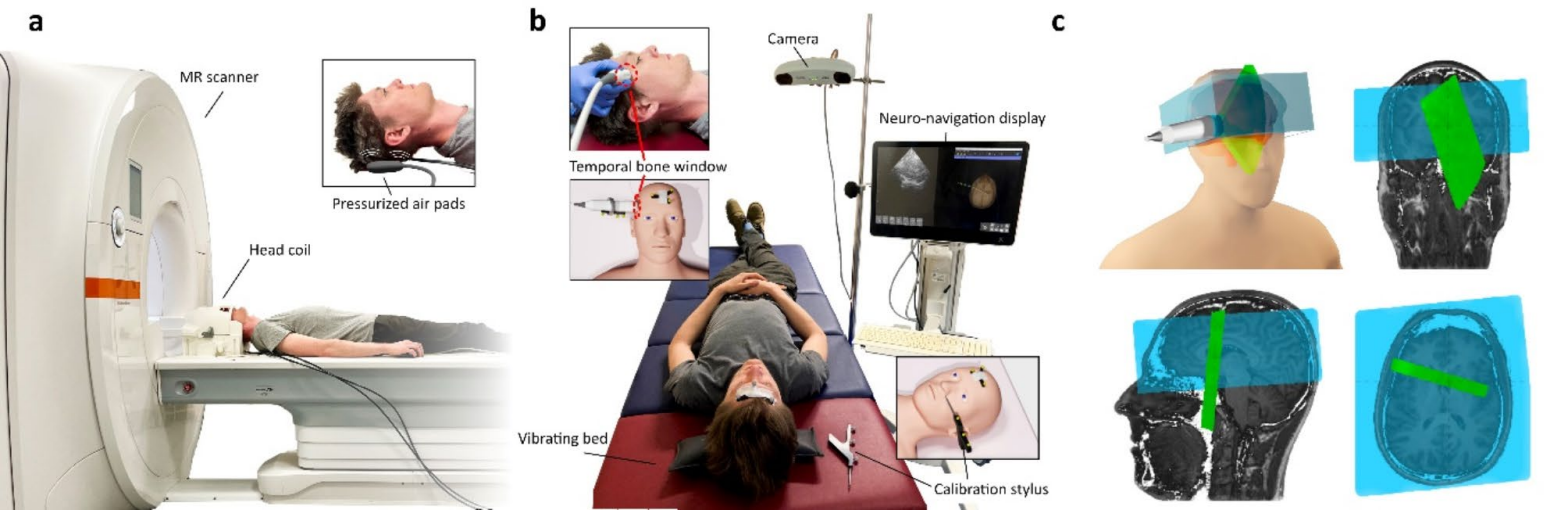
(a) MRE experimental setup. Two pressurized air pads placed underneath the head of the subject induce vibrations in the brain. (b) THE and optical tracking experimental setup. Fiducial markers (spheres) are attached to the head, a stylus, and the ultrasound probe. First, the optical tracking system is calibrated by pointing the stylus at previously chosen anatomical landmarks. Next, THE is performed by vibrating the head of the subject using a patient bed with an integrated speaker. During the THE examination, the ultrasound probe is positioned at the right temples of the subject and the exact position is recorded by the camera and projected onto the rendered and localized T1-weighted MRI map, thus enabling a neuronavigation display. (c) Resulting alignment of the FoVs of MRE (blue) and THE (green). 3D render (upper left corner) and 2D projections onto a T1-weighted MRI map (upper right: coronal view, lower left: sagittal view, lower right: axial view) are included. Written informed consent for publication of their clinical images was obtained from the subject. A copy of the consent form is available for review by the Editor of this journal.
